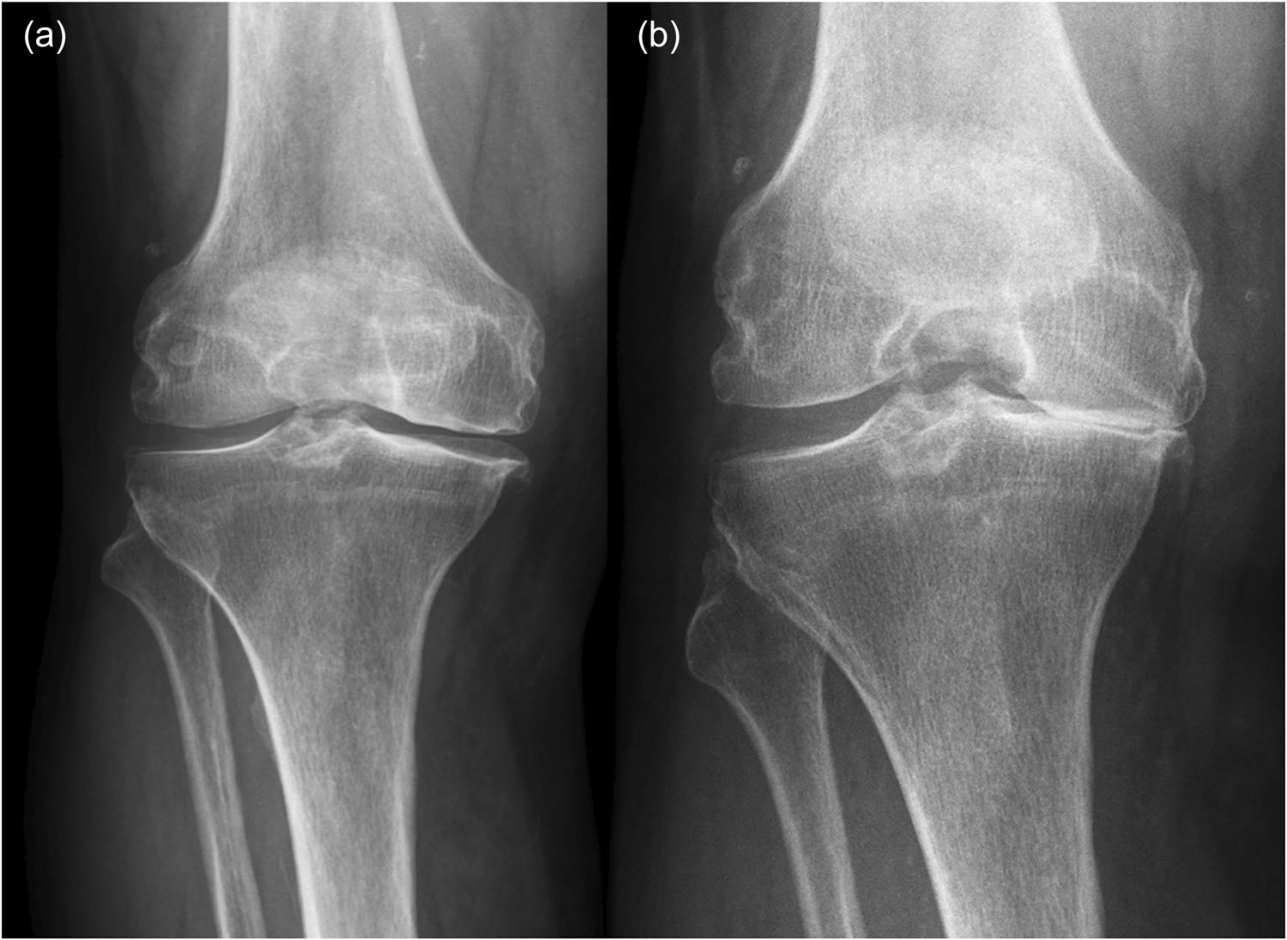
Standard radiographic views used for KL grading: (a) anterior‐posterior (AP) weight‐bearing view and (b) Rosenberg view (45° flexion posteroanterior weight‐bearing view). Both images demonstrate typical positioning for osteoarthritis assessment, with the Rosenberg view providing enhanced visualisation of posterior femoral condyles and tibiofemoral joint spaces.
